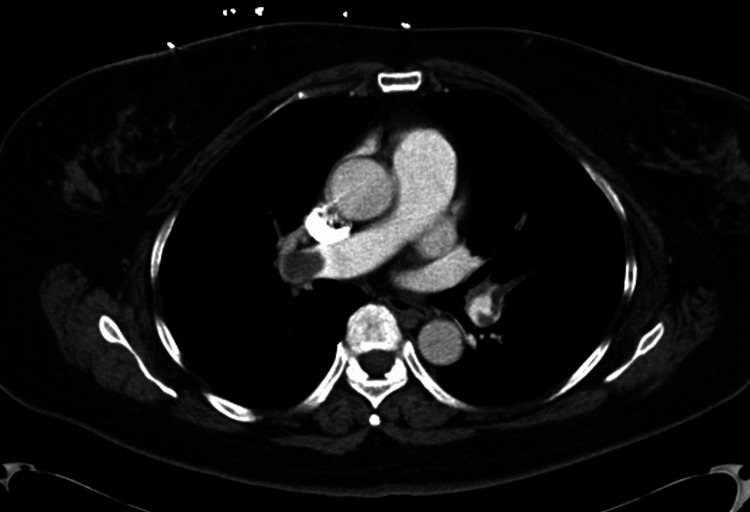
A computed tomography angiogram of the chest (axial images) shows pulmonary embolisms at the distal right pulmonary artery extending into the right interlobar pulmonary artery and segmental branches.
